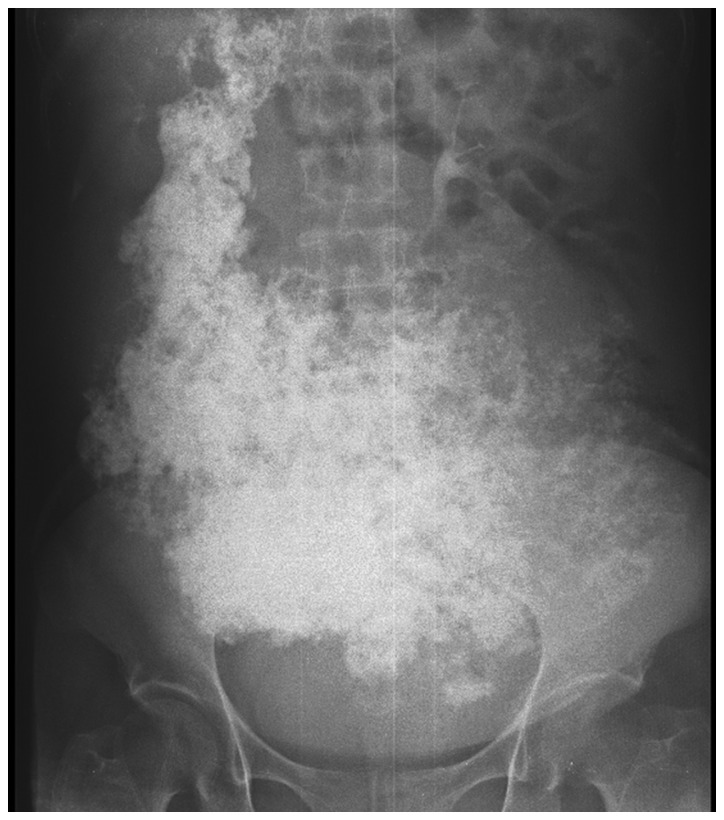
Plain radiography showing extensive calcifications in the abdomen.
